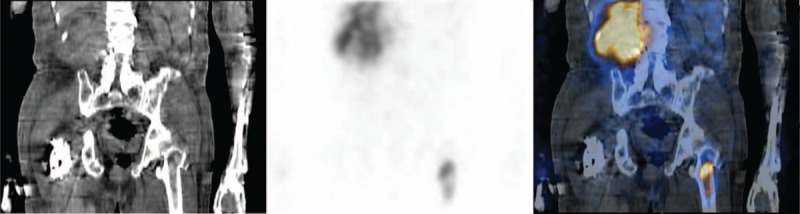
Regional SPECT/CT revealed soft tissue mass with intensely increased Ga-67 uptake at right abdomen and left proximal femur. Discordantly increased Ga-67 compared with Tc-99m MDP activities was noted in the left proximal femur.
